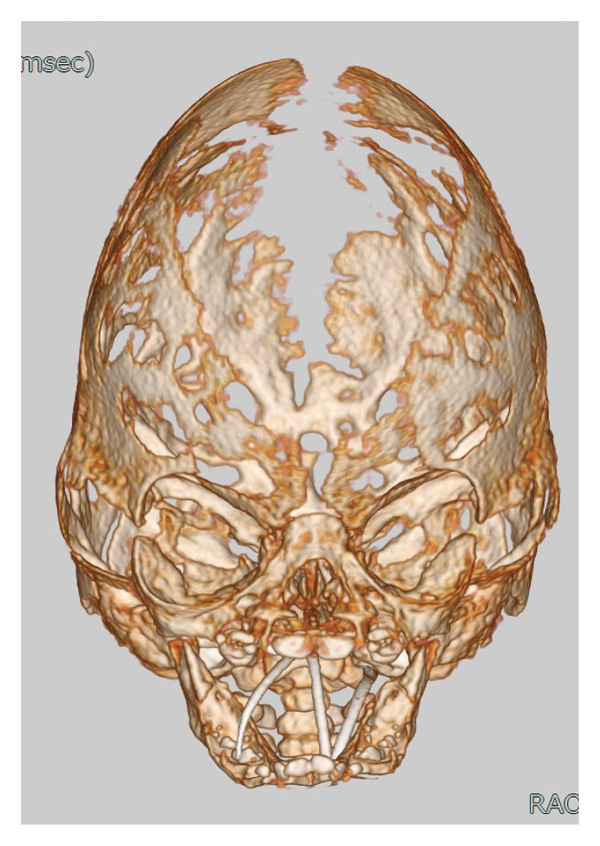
Head three‐dimensional reconstructed CT image (day 26). Bilateral coronal and lambdoid sutures are fused, with a markedly enlarged anterior fontanelle. The skull exhibits a cloverleaf‐like deformity with thinning of the bone, suggesting increased intracranial pressure.
